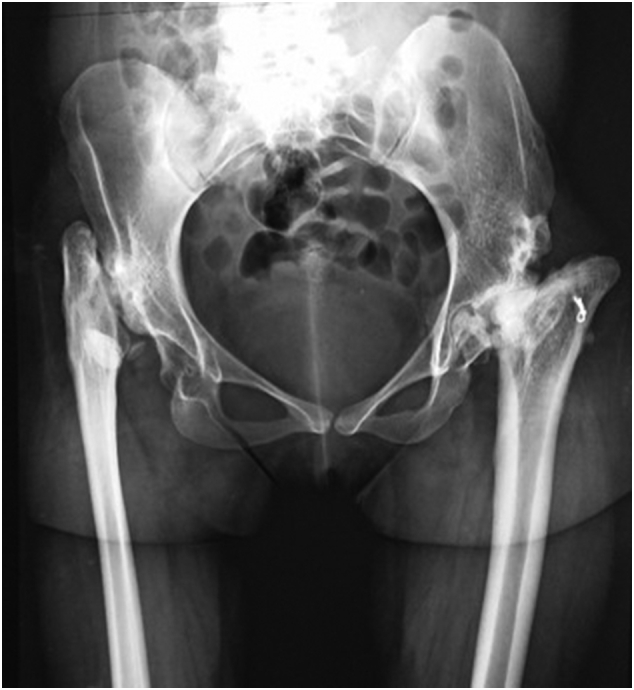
X-ray showing bilateral destruction of the hip joints which was more severe on the right side.
